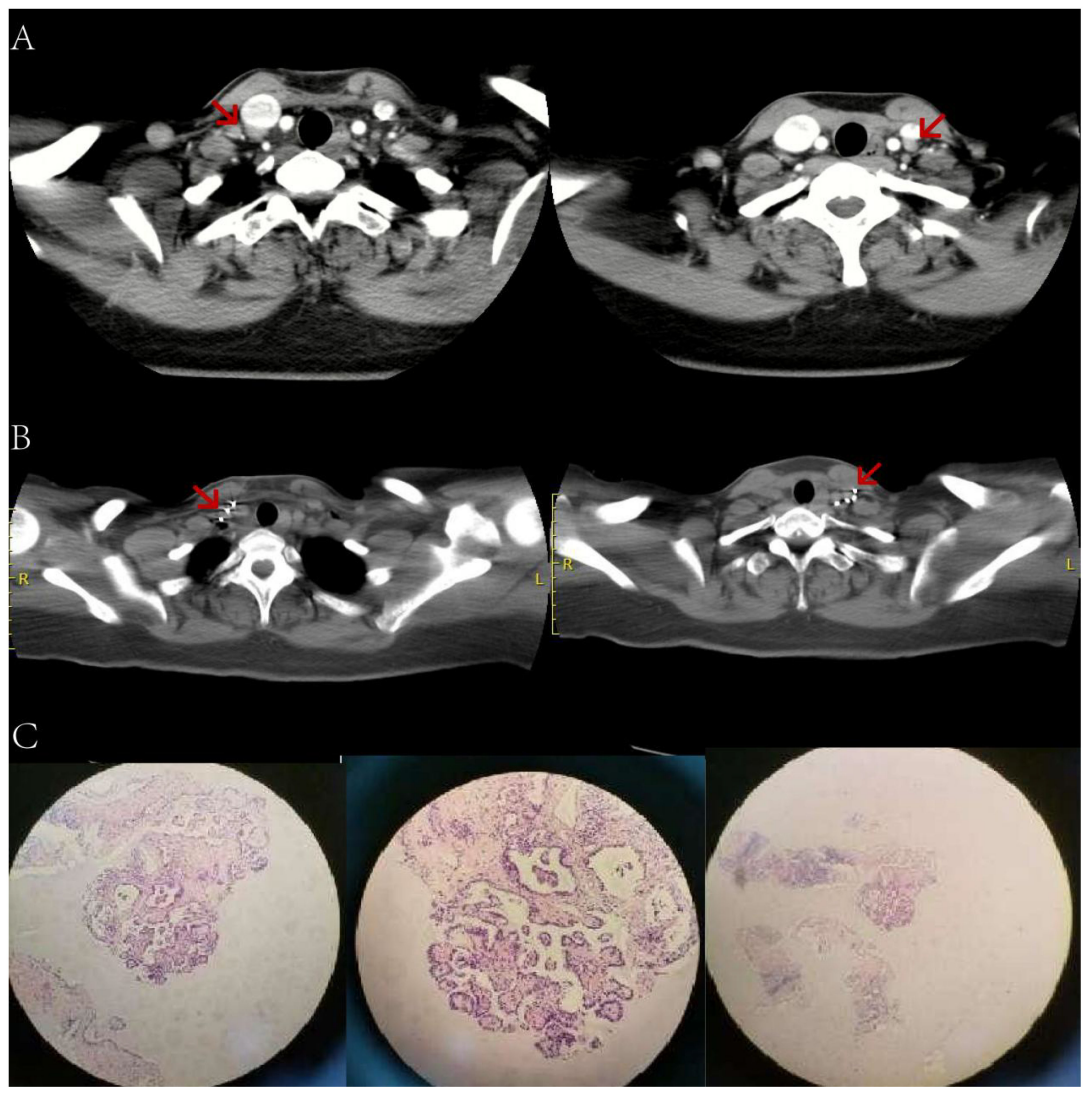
A female patient, aged 55, who underwent thyroid cancer surgery more than 2 years ago. (A) An enhanced CT scan of the neck reveals additional bilateral cervical lymph node metastases (indicated by arrows). The preoperative Tg level was 63.70ng/ml (in a TSH suppressed state). (B) 6 months after receiving 125 iodine radioactive particle implantation treatment, the lesion has significantly reduced in size compared to before. The Tg level is now 3.50ng/ml. (C) Pathology examination shows a small amount of epithelial cells in the tissue, indicating papillary severe heterogeneous hyperplasia. CT, Computed Tomography; Tg, Thyroglobulin; TSH, Thyroid stimulating hormone.
